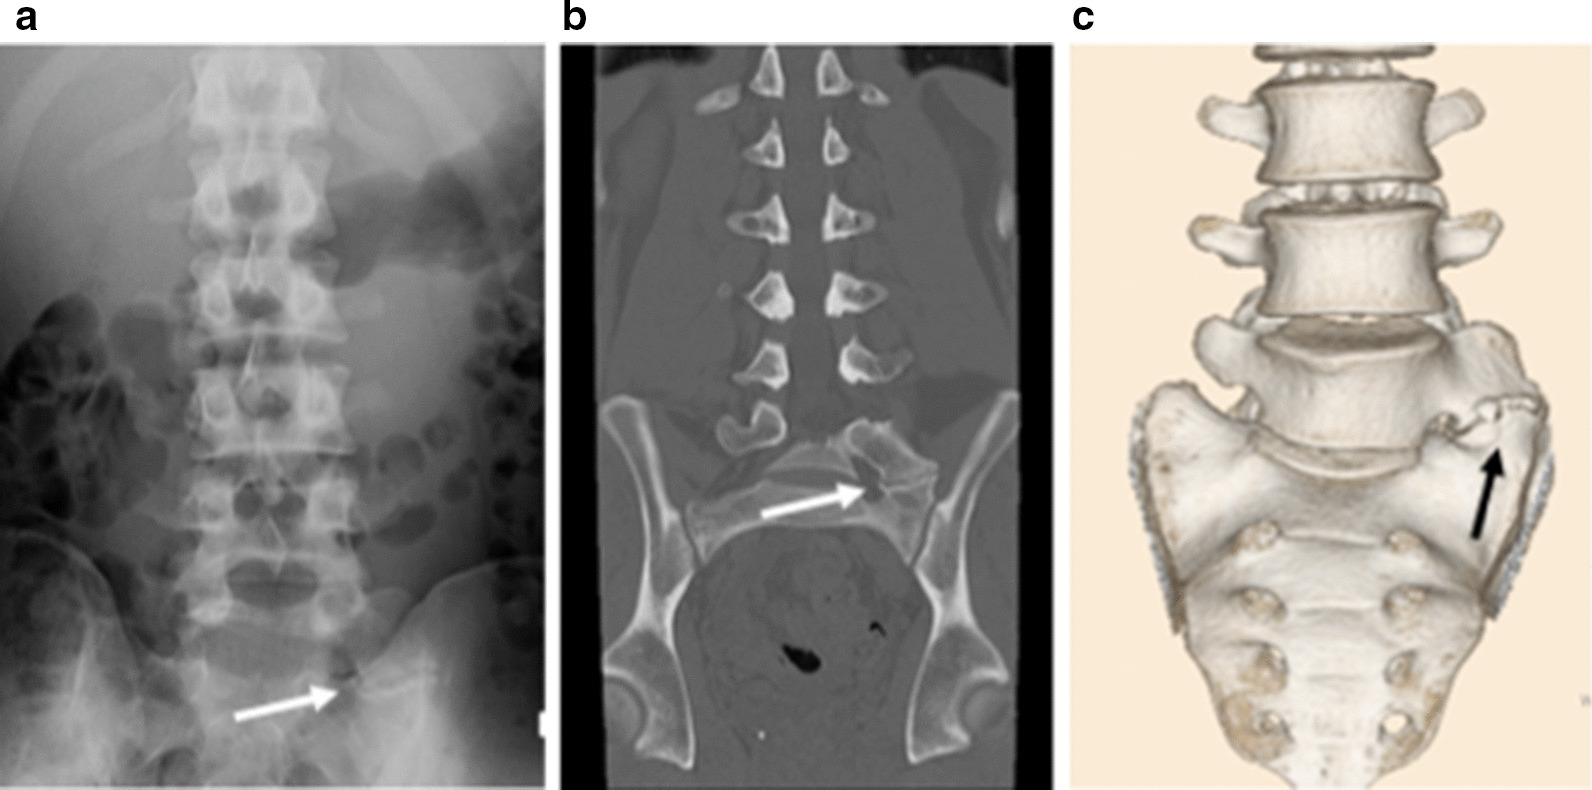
a Preoperative anteroposterior plain radiography. b Preoperative coronal computed tomography (CT) image. c Preoperative three-dimensional (3D) CT image. Arrow indicates pseudoarticulation at the left side.
